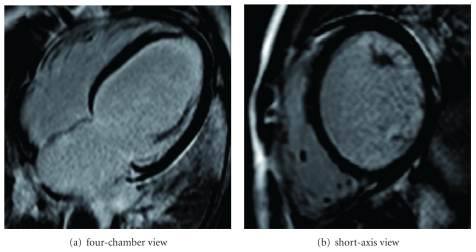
Epicardial late enhancement in the basal inferolateral region in cardiac MRI.
